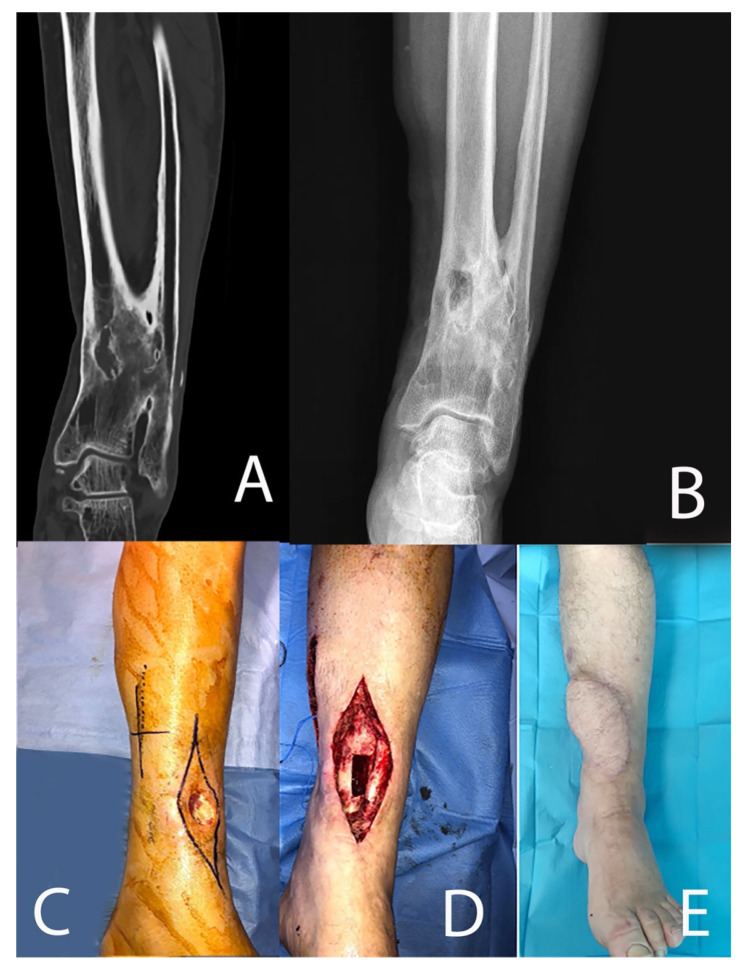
Chronic osteomyelitis of the distal tibia ((A): pre-operative coronal view CT scan) treated with curettage and antibiotic-loaded beads ((B): anteroposterior X-rays). The discharging sinus (C) was excised together with infected soft tissues (D) and reconstructed with a free flap ((E): 4 months follow-up).
